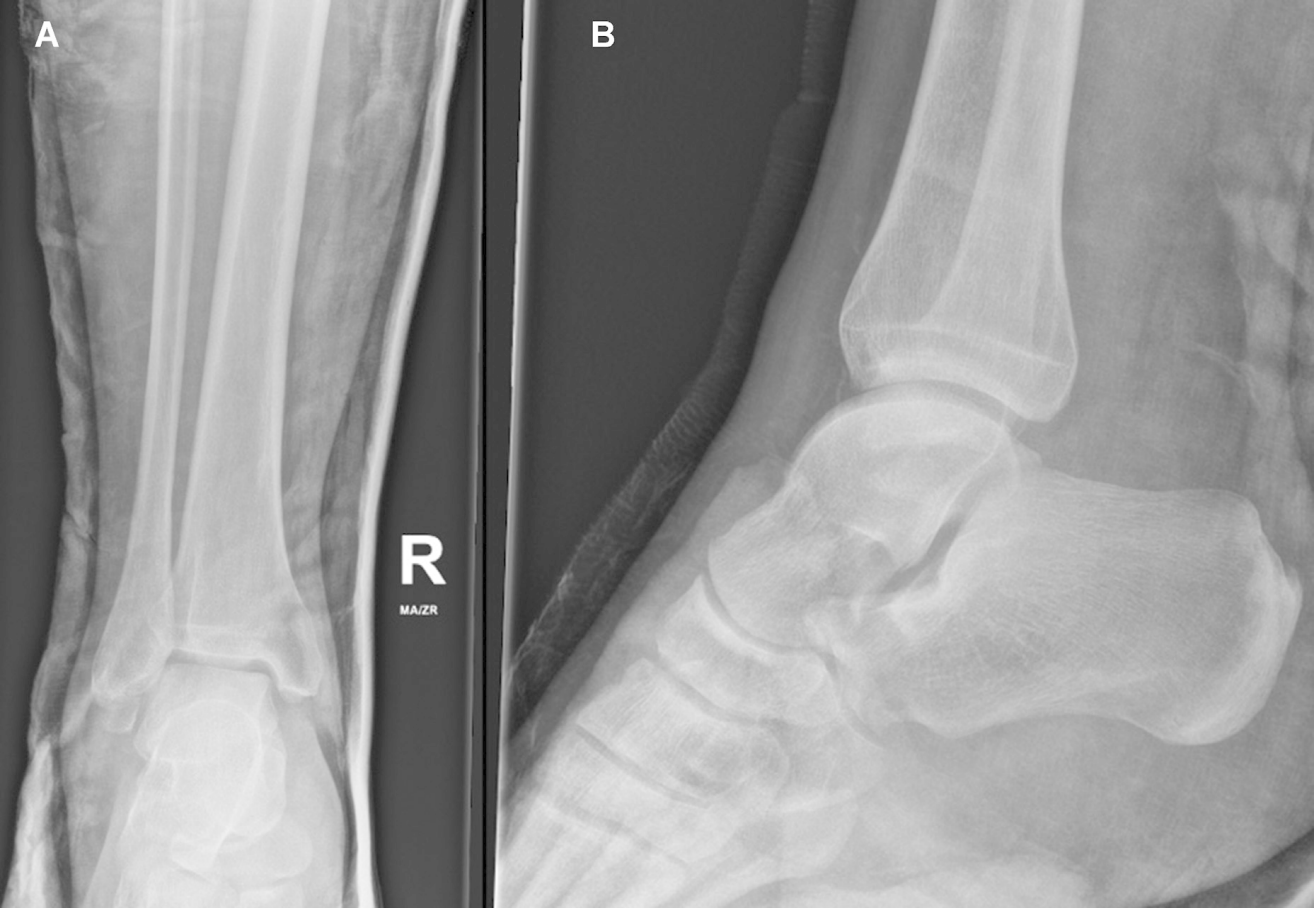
Postreduction anteroposterior (A) and lateral (B) radiographs of the right ankle. There is satisfactory alignment at the talonavicular, subtalar, and tibiotalar joints.
